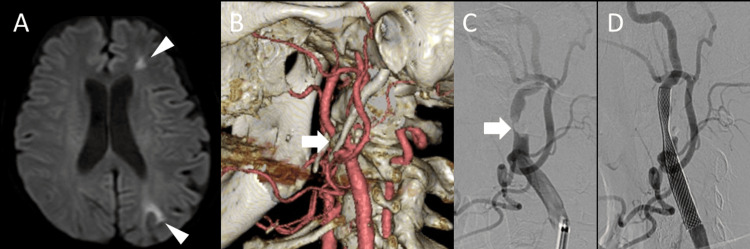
Imaging findings before and after the initial carotid artery stenting. (A) Diffusion-weighted MRI showing cerebral infarction in the left watershed area (arrowheads). (B) Three-dimensional CT angiography (3D-CTA) revealing carotid artery stenosis with surrounding calcification at the C2 vertebral level (arrow). (C) Digital subtraction angiography (DSA) of the left common carotid artery demonstrating severe stenosis (95% by the North American Symptomatic Carotid Endarterectomy Trial (NASCET) criteria) of the left internal carotid artery (arrow). (D) Post-stenting DSA showing improvement of stenosis to 39% by the NASCET criteria.
